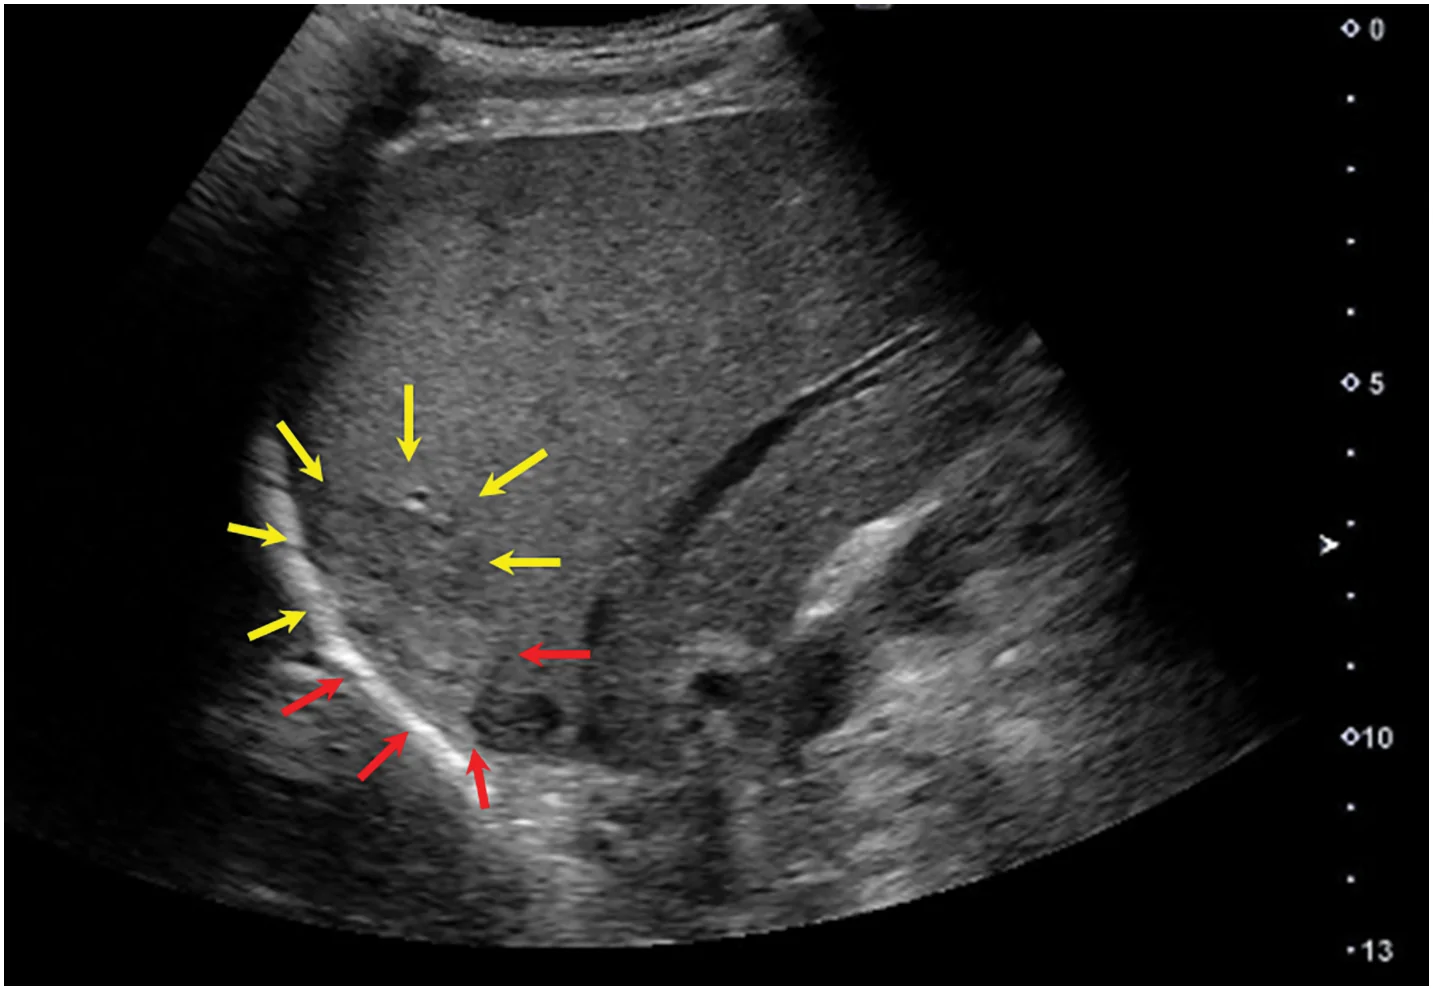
Preoperative AUS. AUS reveals a well-demarcated, dumbbell-shaped mass (maximum diameter, 35 mm) in the posterior segment of the liver. The inner portion appears slightly hyperechoic (red arrows), whereas the outer portion shows isoechoic to hypoechoic features (yellow arrows). AUS, abdominal ultrasonography
